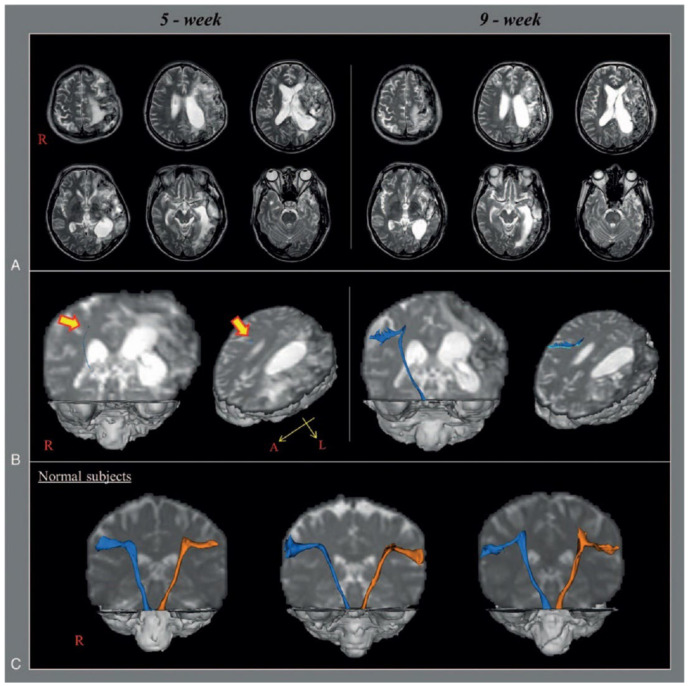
Anatomical structure of CBT captured using DTT [33]. (A) T2-weighted brain magnetic resonance (MR) images at 5 and 9 weeks after onset show leukomalactic lesions in left middle cerebral artery territory. (B) Diffusion tensor tractography (DTT) for the corticobulbar tract (CBT). On 5-week DTT, the right CBT is discontinued at the subcortical white matter and shows severe narrowing compared with those of normal subjects, whereas the left CBT is not reconstructed. By contrast, on 9-week DTT, the right CBT is extended to the cerebral cortex with thickening, whereas the left CBT is still not reconstructed. (C) Reconstructed CBT of normal subjects (55-year-old man, 57-year-old man, and 60-year-old man), blue arrow: right CBT, orange arrow: left CBT. Copyright by Jang et al. (2017) [33].
